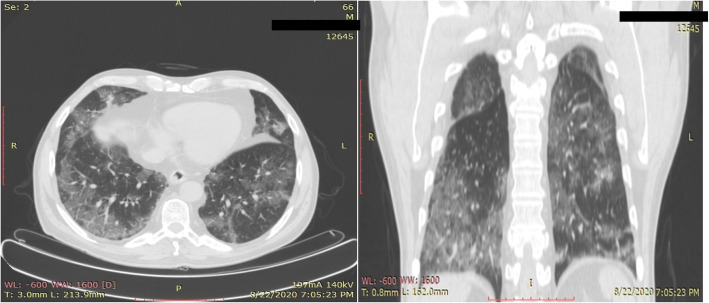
Chest CT of a 66-year-old male patient showing bilateral ground glass pulmonary changes predominantly in peripheral distribution. The lung involvement was 50–75% and the CT severity index score was 4. The PCR test for COVID-19 was positive for this patient
